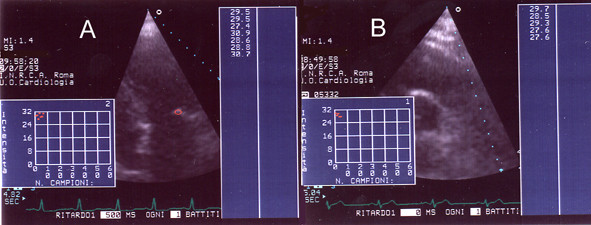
Score 2. Panel A: AD of mitral valve (apical scan); Panel B: AD of aortic valve (parasternal scan: short axis).
